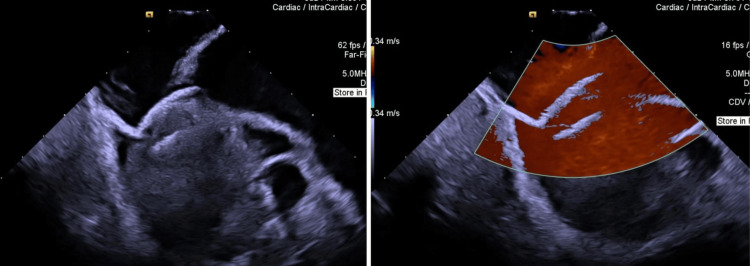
Intracardiac echocardiography (ICE) guided patent foramen ovale (PFO) closure The left panel demonstrates the PFO closure device during deployment. The right panel shows post deployment colour Doppler with complete abolition of the right to left interatrial shunt and stable device positioning.
